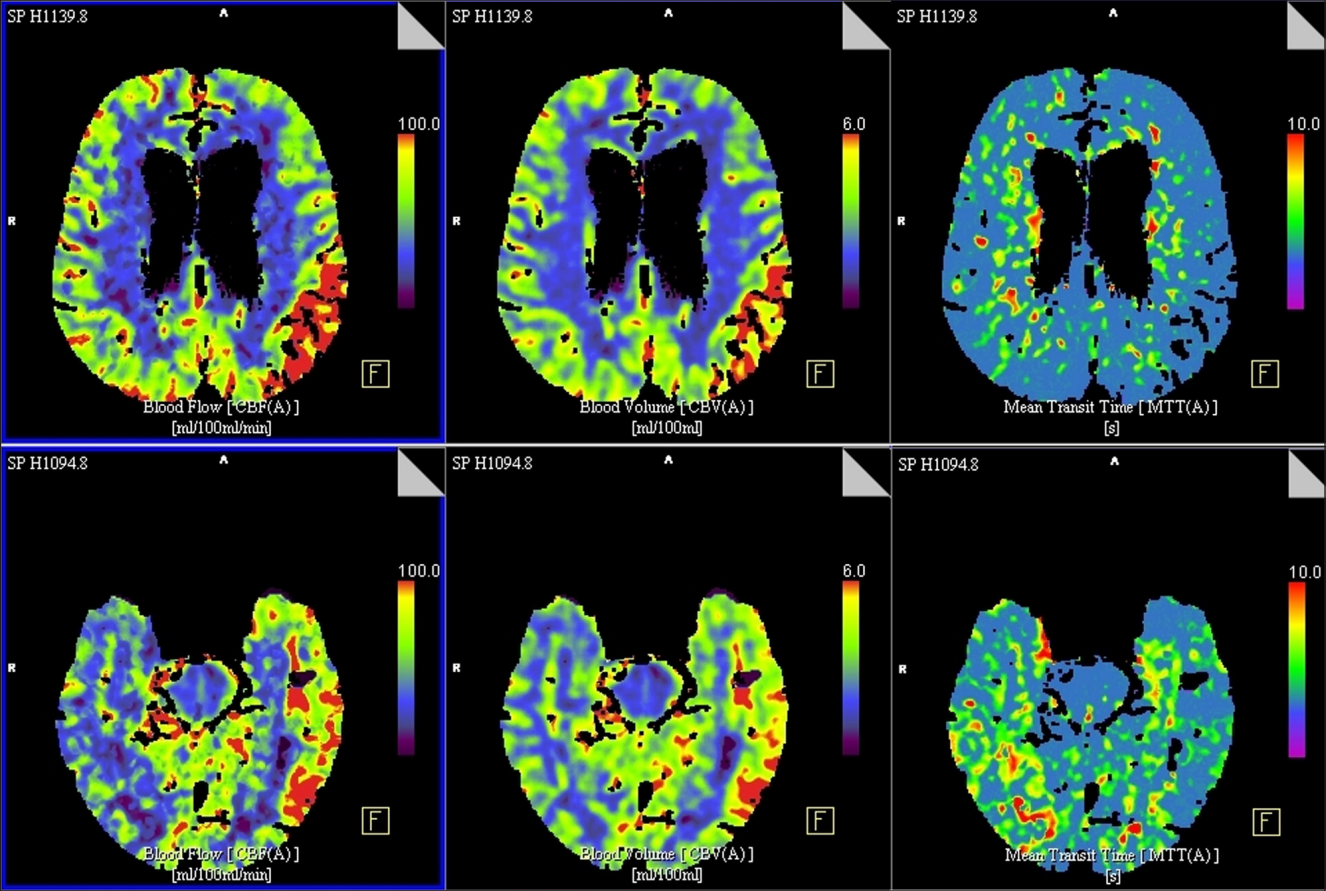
Top (a) and bottom (b) slices of brain perfusion CT shows extensive perfusion alterations in the entire left parietotemporal region, consisting of a normal to slightly diminished MTT (right column), and an increased CBF (left column) and CBV (middle column).
